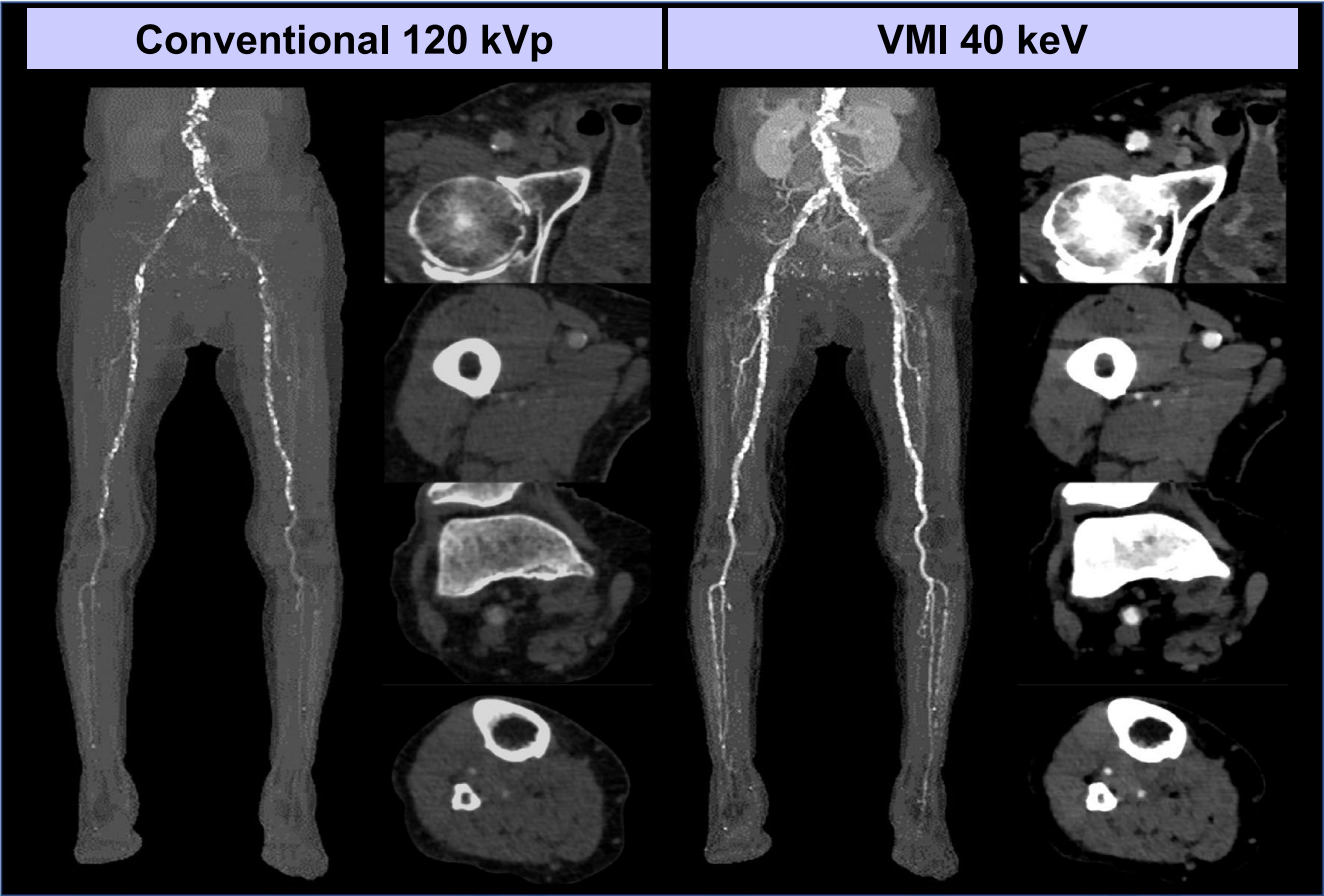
A 91-year-old woman with toe gangrene underwent runoff CTA using dual-layer detector scanner. A total of 25 mL of ICM (370 mgI/mL, diluted with 50 mL normal saline) was injected over 25 s. Conventional imaging at 120 kVp demonstrated insufficient arterial opacity, while VMI at 40 keV showed improved visibility of the lower extremity arteries
